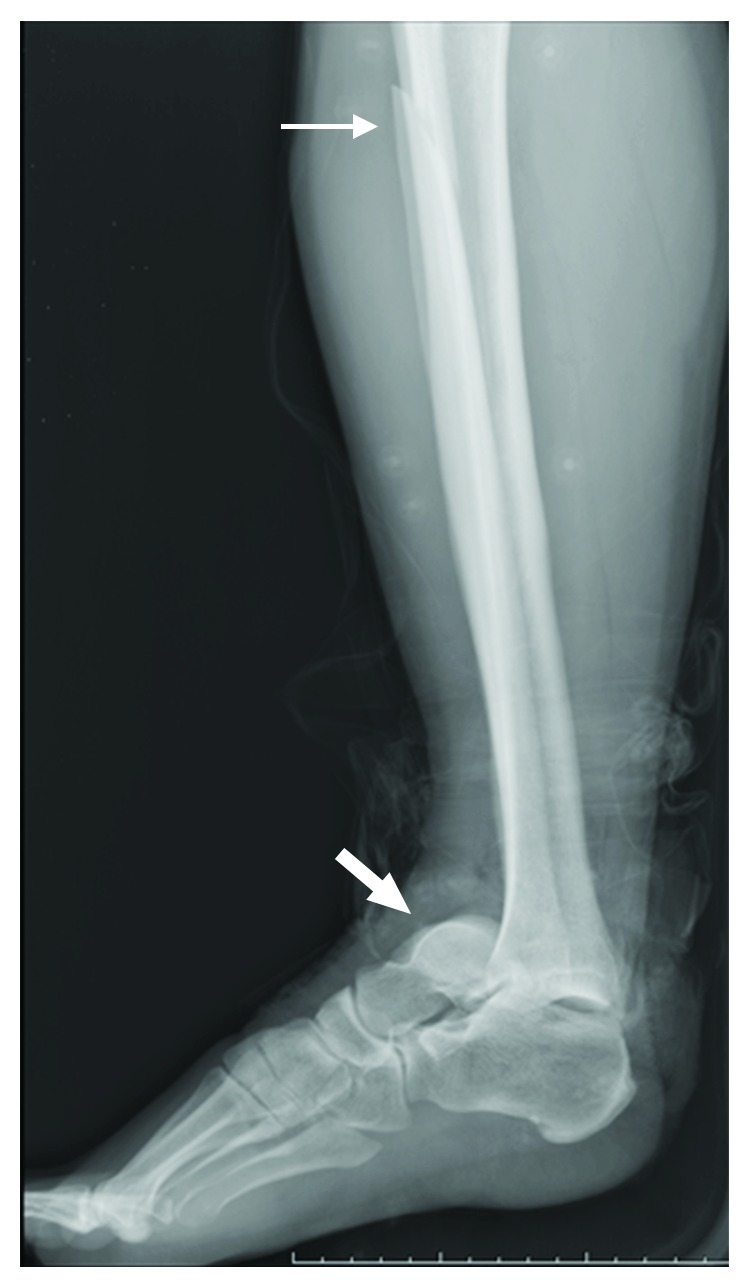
Lateral radiograph of the right shin and foot. Anterior dislocation of the talus (thick white arrow) and proximal fibular fracture (thin white arrow) are seen.
